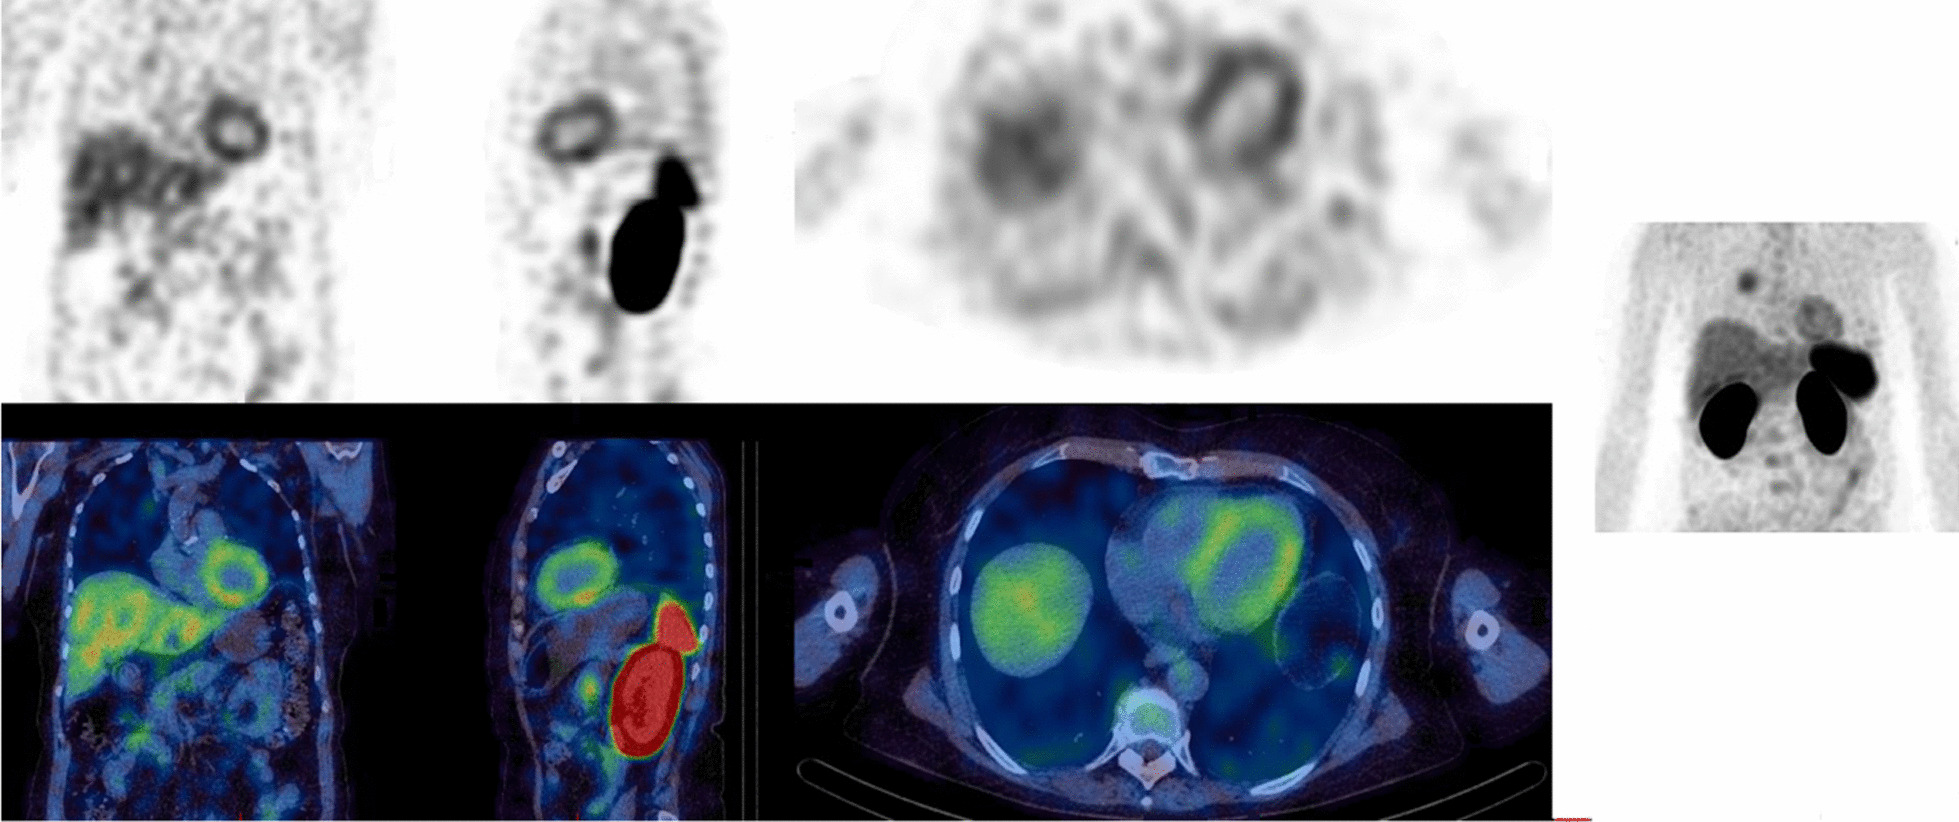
Baseline [^99m^Tc]NM-01 SPECT/CT images for a patient with non-small cell lung cancer. In addition to the uptake of [^99m^Tc]-labelled anti-PD-L1 single-domain antibody (NM-01) in the right lung tumour, tracer uptake is seen in the myocardium. The left ventricular: blood pool maximum region of interest (ROI_max_) ratio was 3.1, and the right ventricular: blood pool ratio was 1.5. In addition, there is a normal distribution of hepatic, bone marrow, splenic and renal activity. Very low muscle activity is present in the paraspinal muscles, in contrast to the increased myocardial PD-L1 expression
